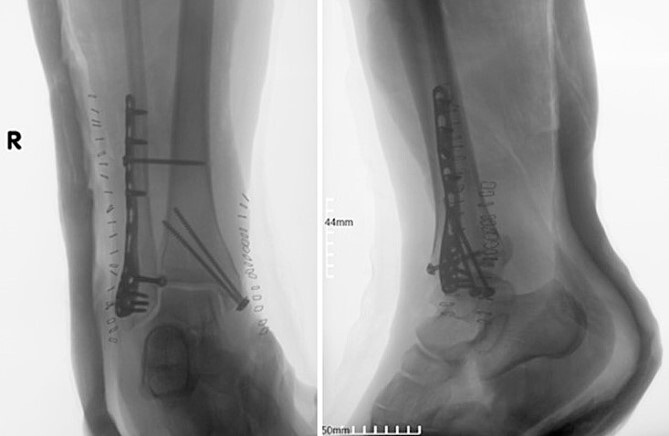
Postoperative anteroposterior and lateral X-ray of the ankle joint after ORIF of the fracture.
